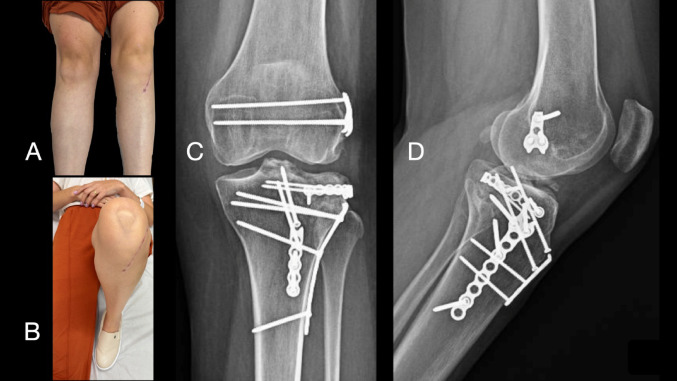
A, B Clinical photographs at 29 months postoperatively demonstrating full knee range of motion. C, D Anteroposterior and lateral radiographs of the left knee showing fracture healing in an appropriate alignment
